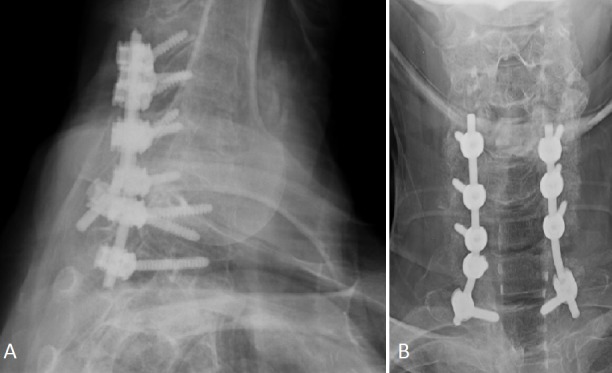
X-rays of Cervical Spine A) Lateral X-ray; B) AP X-ray showing postoperative images of instrumentation.
